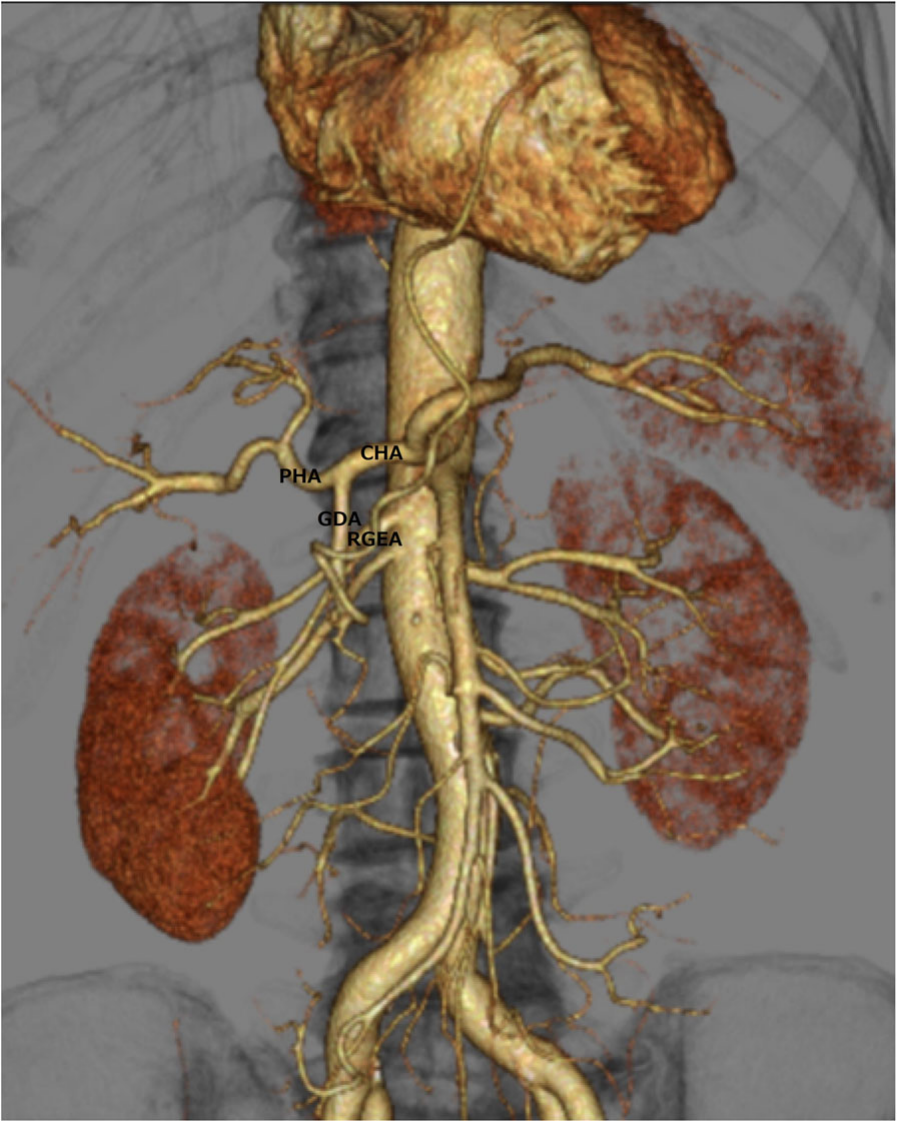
3D-CTA revealed that the gastric tube was the feeding vessel to RGEA branching from GDA
